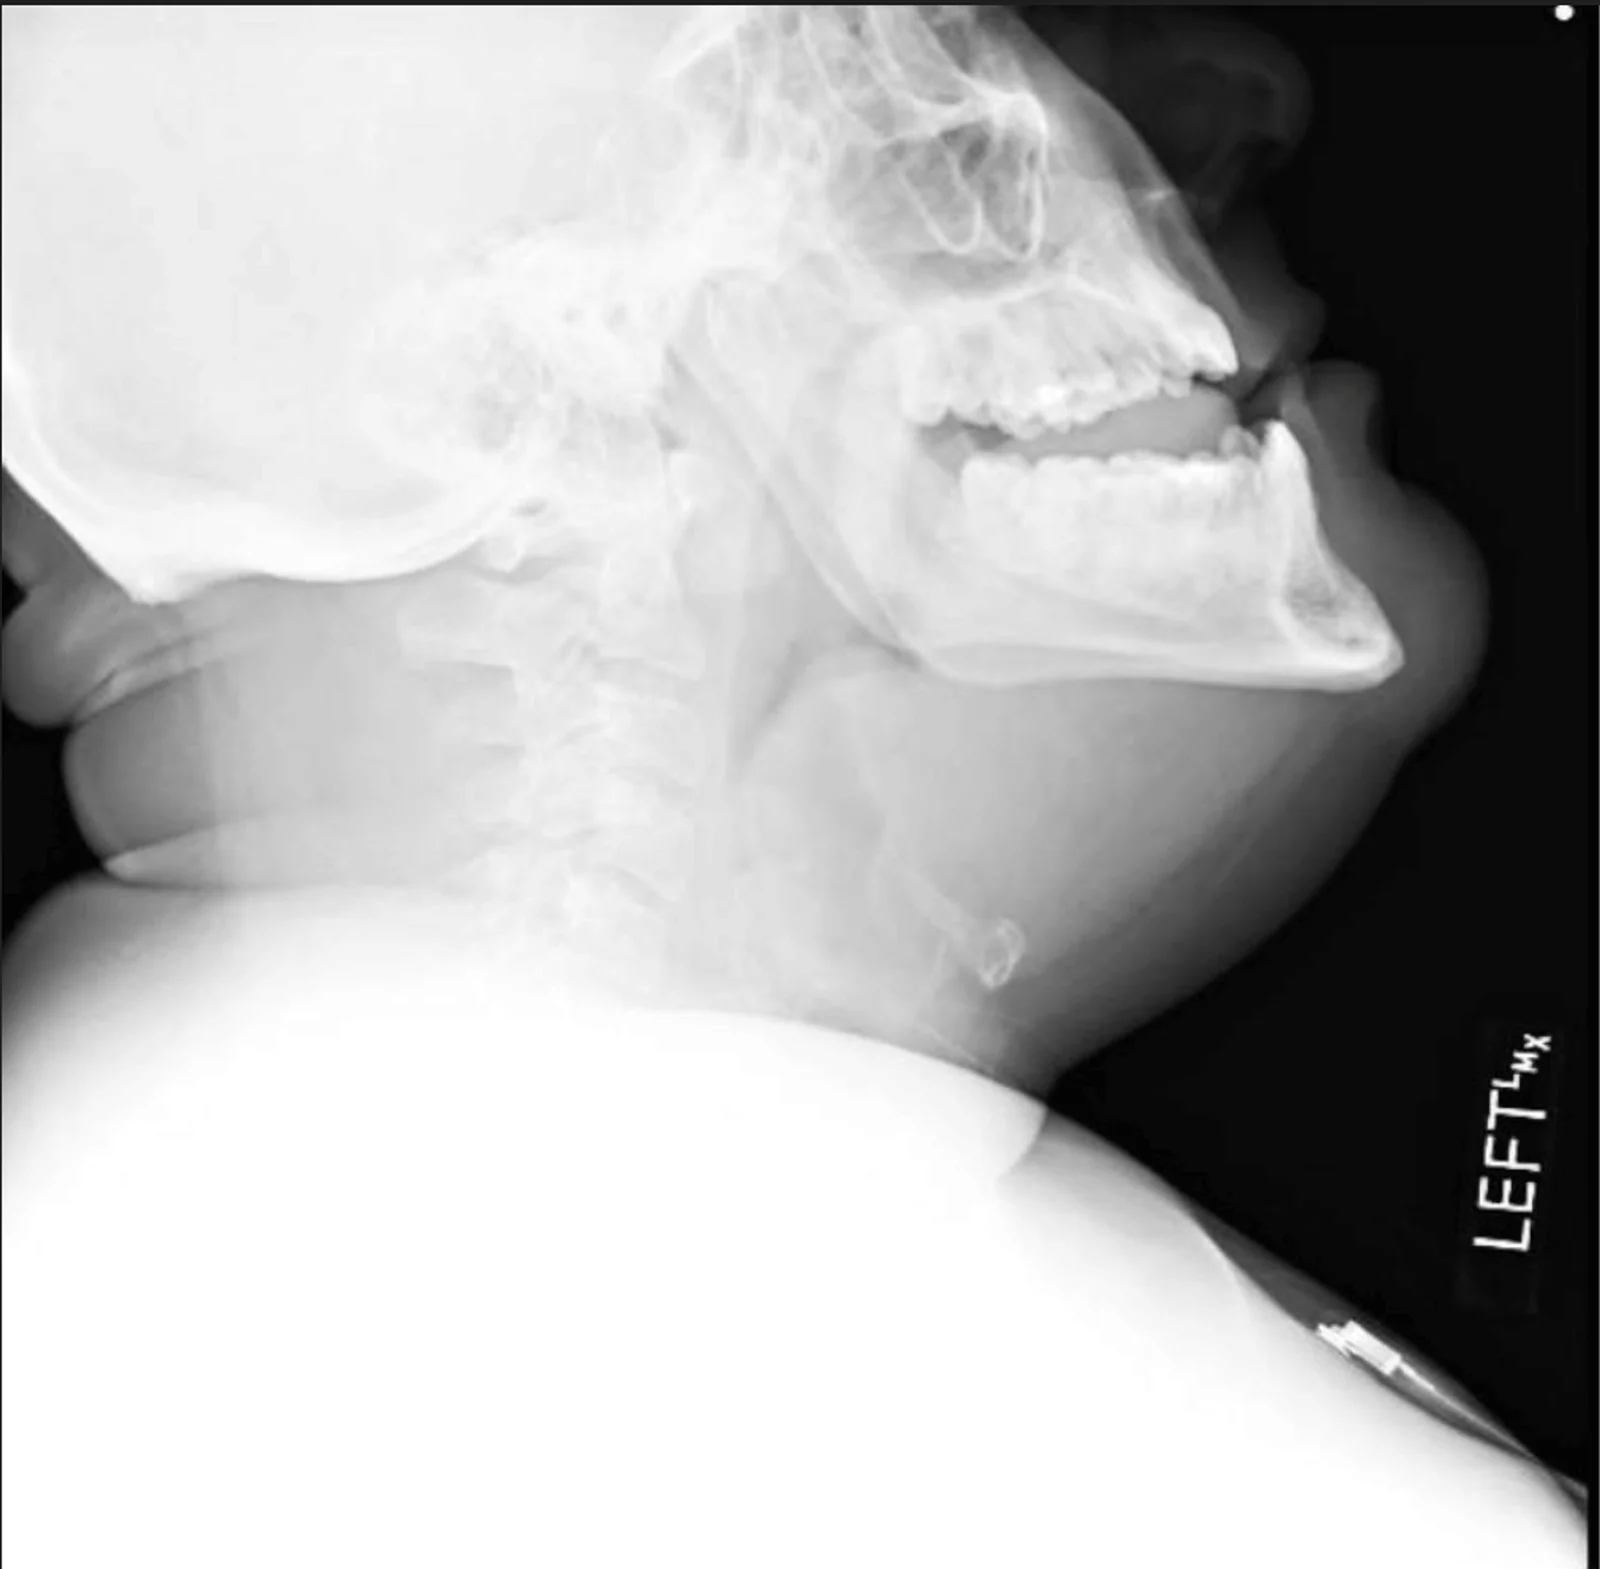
Lateral soft tissue neck radiograph at presentation Soft tissue edema is present in the submandibular region, but the radiologist reported the study as markedly limited by the patient's body habitus, with no further detail obtainable. The depth of the anterior cervical soft tissues, which accounts for the limited yield, is itself visible.
